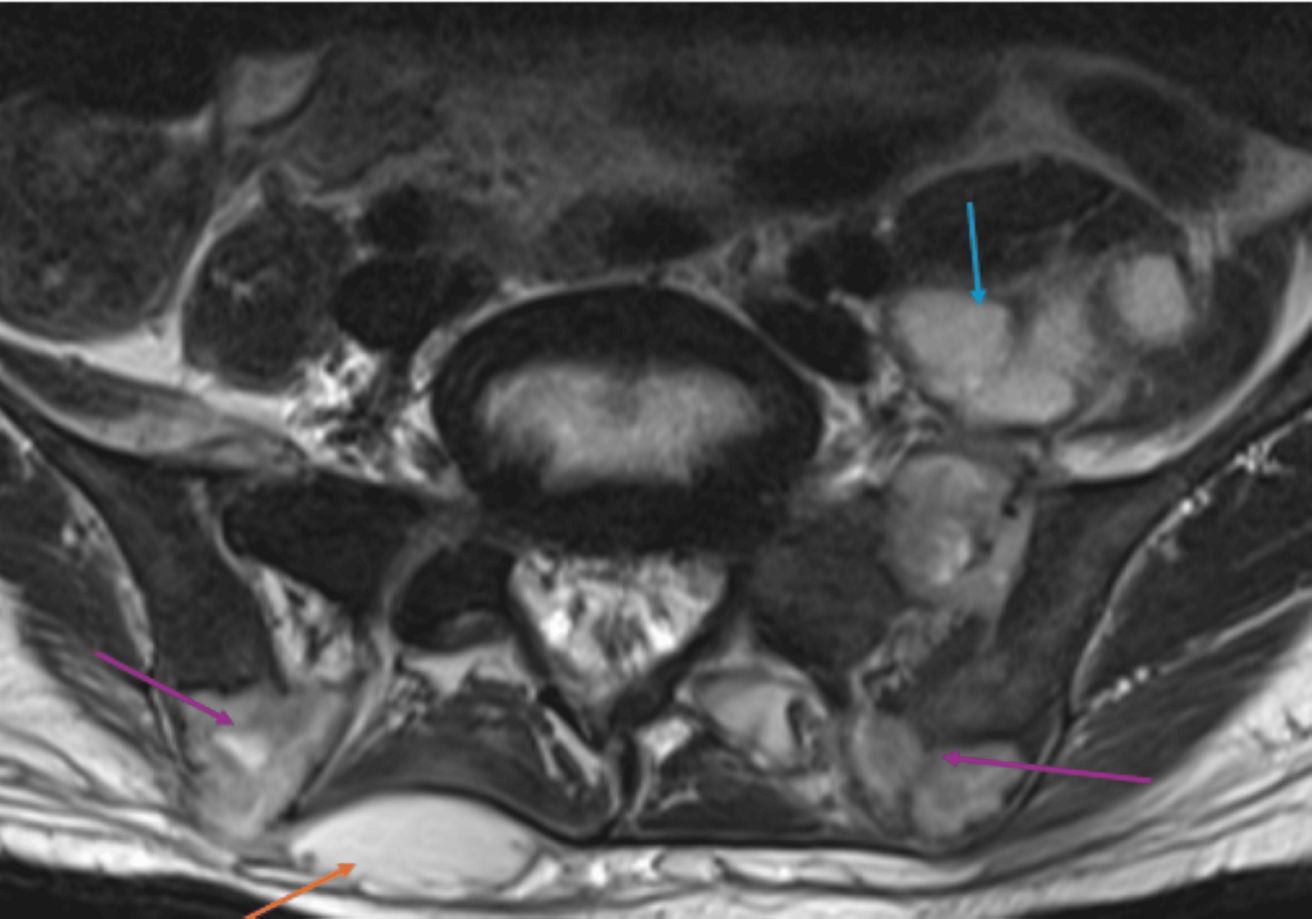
Axial turbo T2-weighted MR image of the upper pelvis demonstrating bilateral iliac bone lesions (purple arrows), left iliopsoas abscess (blue arrow), and posterior subcutaneous abscess (orange arrow) MR: magnetic resonance
